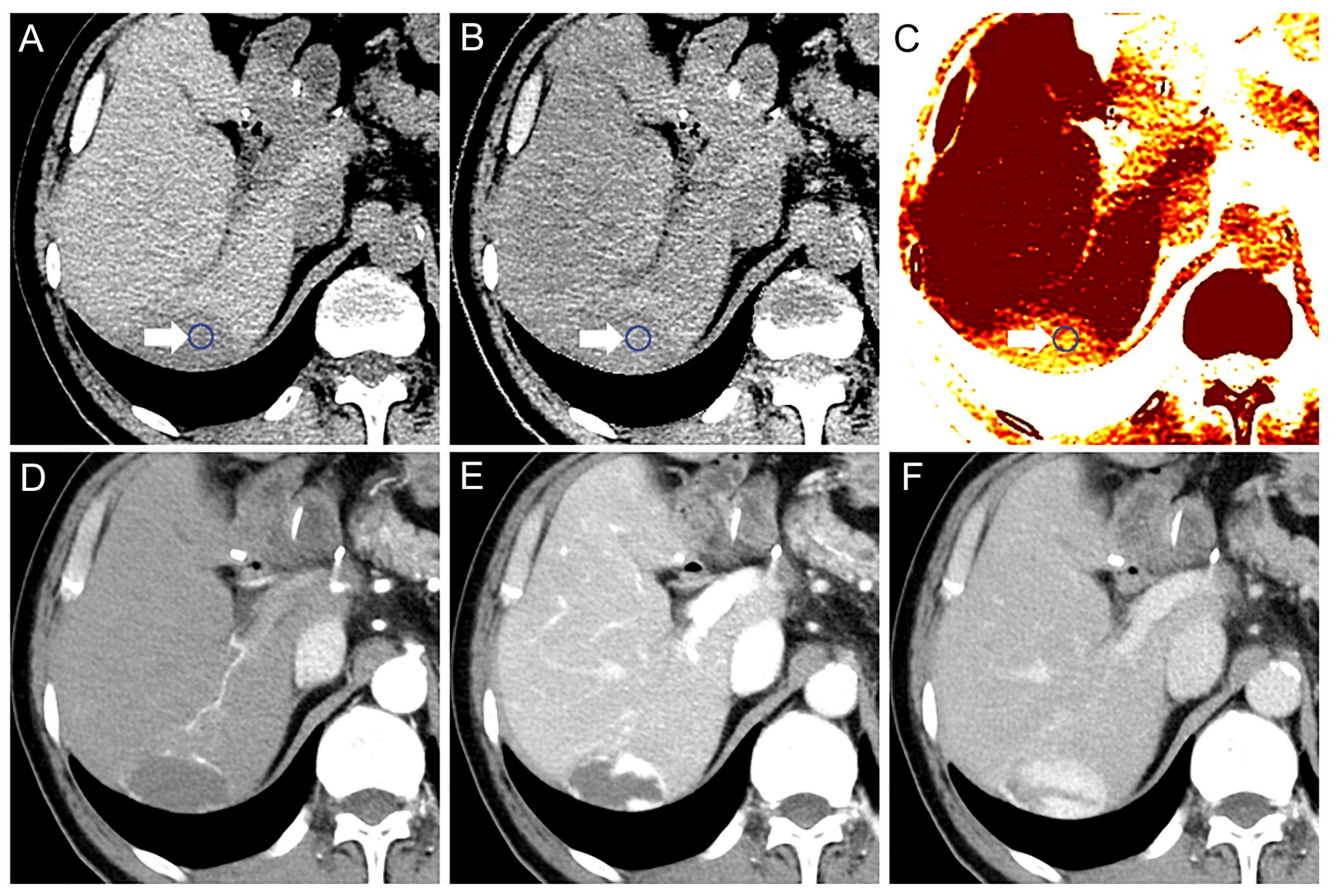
Representative case A: Unenhanced CT scan image; B: Zeff image; C:  Liver fat image; D: CE CT scan image late arterial phase; E: CE CT scan image portal venous phase; F: CE CT scan image equilibrium phase The lesion was characterized as a hepatic hemangioma. The corrected Zeff was calculated to eliminate the effect of fat. The white arrows point at the ROI, which was set in the area with no necrosis. The corrected Zeff was 7.622. CE: Contrast-enhanced, ROI: Region of interest
